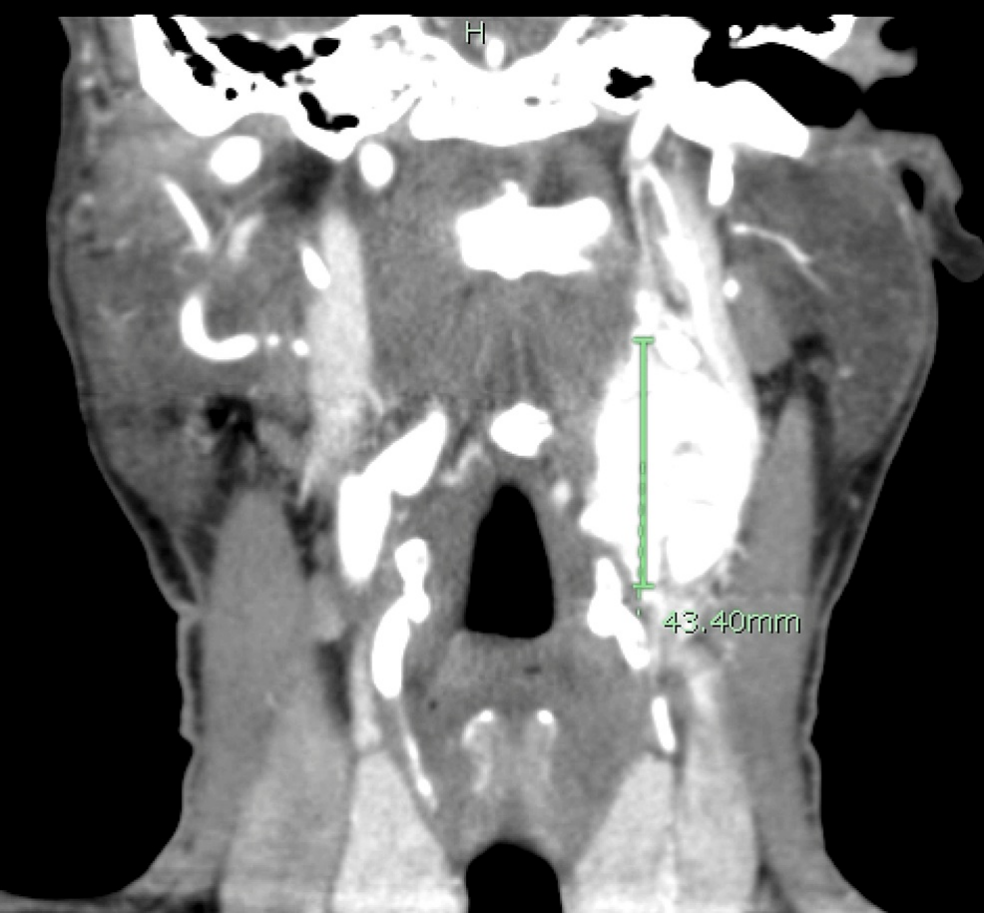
Coronal section of a neck CTA showing a left neck mass measuring 4.3 cm craniocaudal measurement
